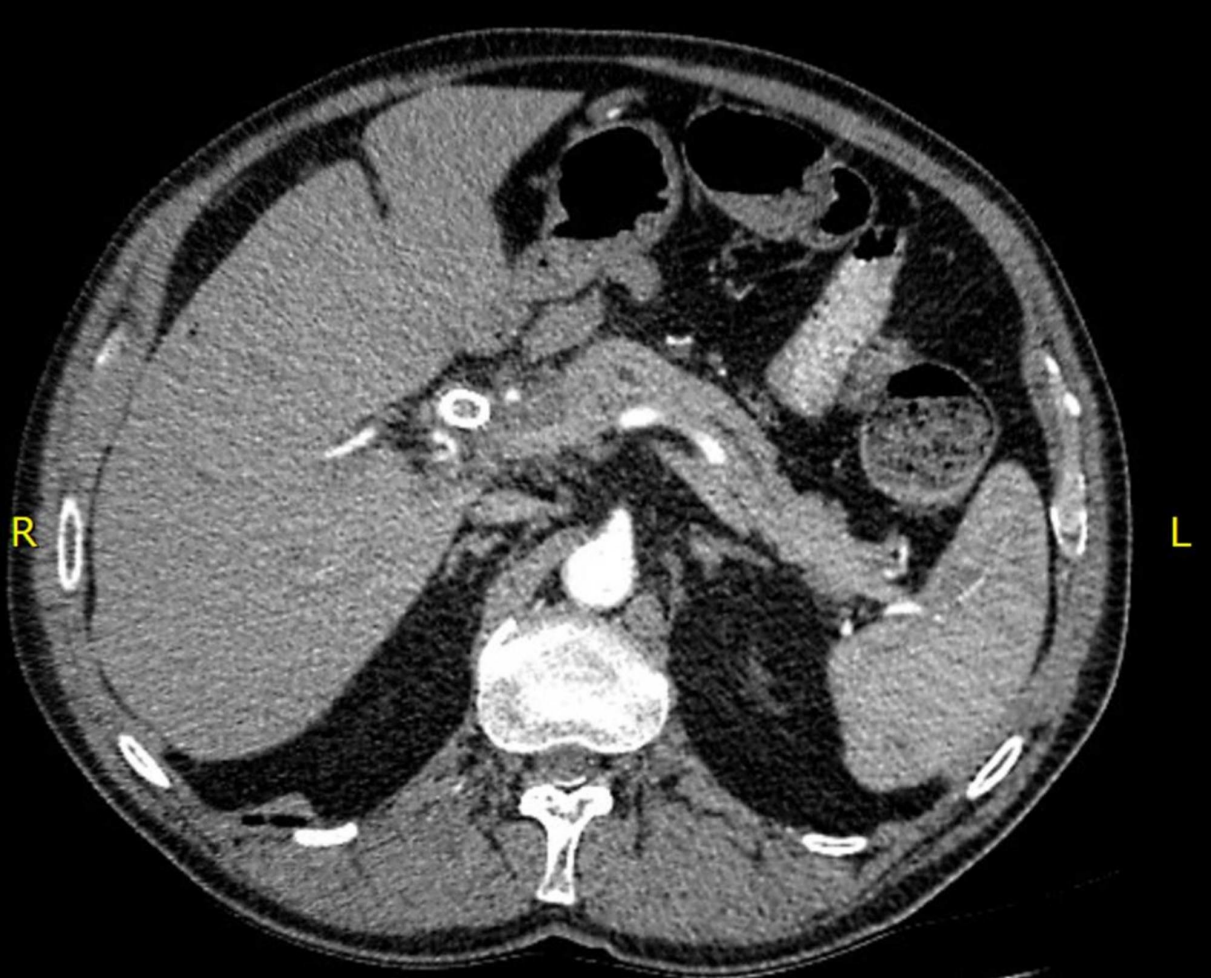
CT scan of the abdomen from three months post-SBRT to the right upper lobe lung adenocarcinoma (NSCLC) demonstrating the complete resolution of the liver metastasis from the cholangiocarcinoma.
